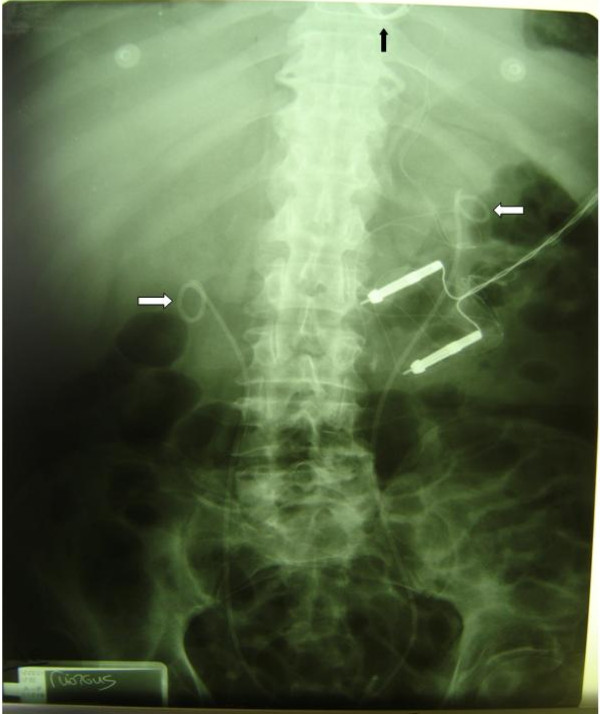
An x-ray performed during the early postoperative period. The ureteral catheters are showing (white arrows) while the annulus of the mechanical valves (black arrow) and the wires of the epicardial pace maker are also seen.
